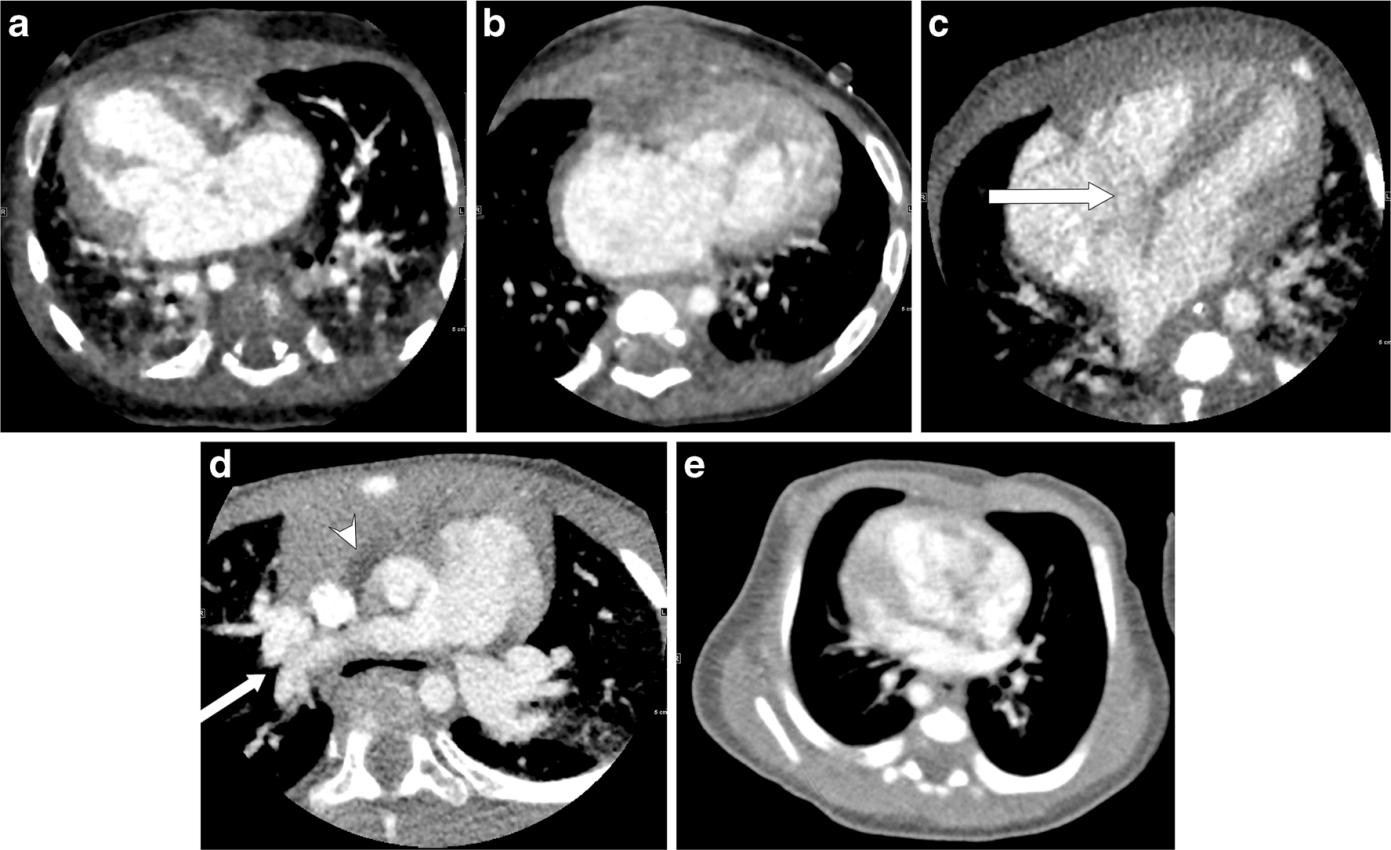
Representative axial contrast-enhanced CT images of the five levels of overall image quality. a Score 5, excellent anatomical clarity and image quality, in a 2-month-old girl with atrial septal defects and ventricular septal defect. b Score 4, good anatomical clarity and image quality with minor motion artifacts, in a 6-month-old boy with hypoplastic left heart syndrome after Norwood procedure and pulmonary angioplasty. c Score 3, fair image quality with motion artifacts extending less than 5 mm from the vessel center *arrow*, here in a 5-day-old boy with transposition of the great arteries, atrial septal defect and patent ductus arteriosus. d Score 2, poor image quality, inadequate delineation between the vessel and surrounding tissue *arrow*, presence of streak artifacts extending at least 5 mm from the center of the vessel *arrowhead*, and no useful information obtained. This image is in a 2-year-old girl with atrial septal defect. e Score 1, non-diagnostic image quality, in a 1-month-old girl with transposition of the great arteries
